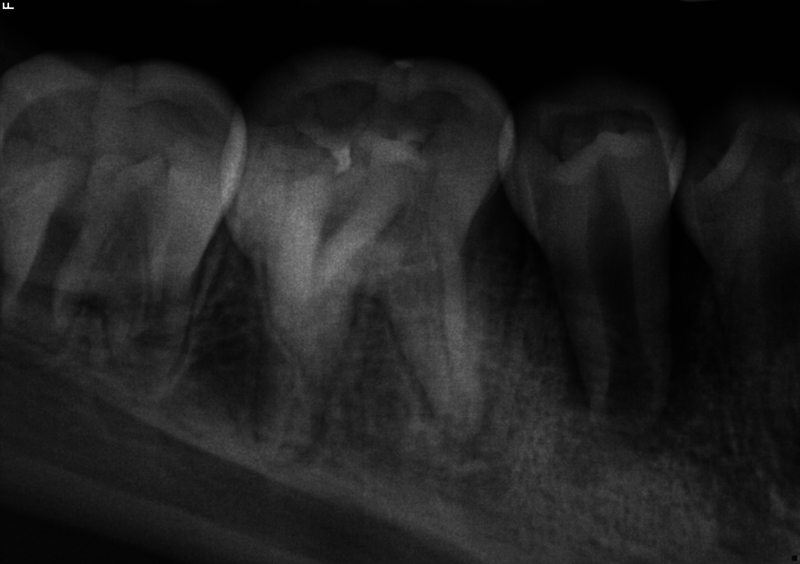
Preoperative periapical radiograph of tooth #29 distally shifted taken at the University of North Carolina at Chapel Hill Adams School of Dentistry.
